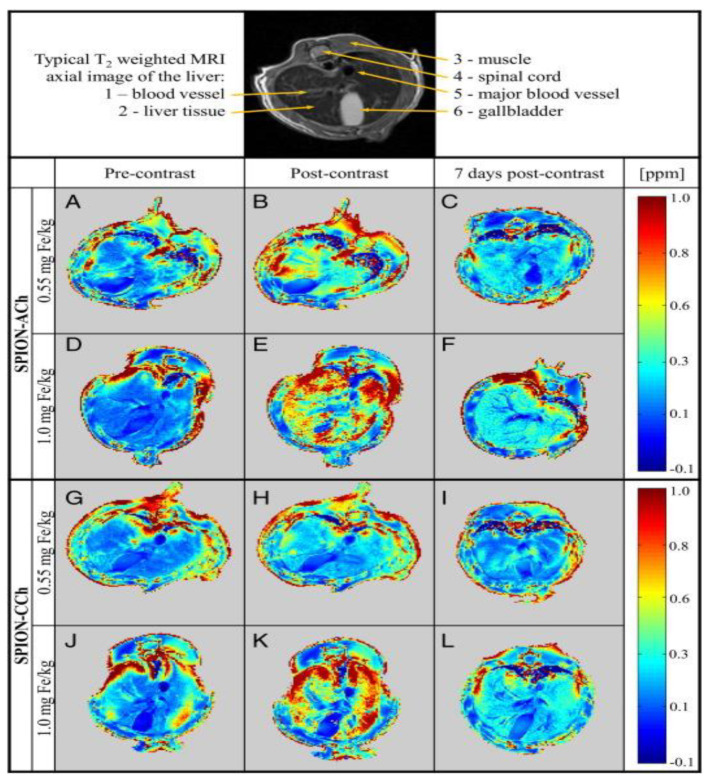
An overview of MNPs’ impact in MRI: The paramagnetic properties of various organs are detected effectively through MRI. The use of MNPs enhances image contrast significantly, but this improvement diminishes within a week, returning to baseline levels. Panels (A,D,G,J) are images taken at pre-contrast with different dosages of SPIONs. Panels (B,E,H,K) are images taken at post-contrast with different dosages of SPIONs. Panels (C,F,I,L) are images taken 7 days post-contrast with different dosages of SPIONs. Reprinted from [47], with permission from Elsevier.
